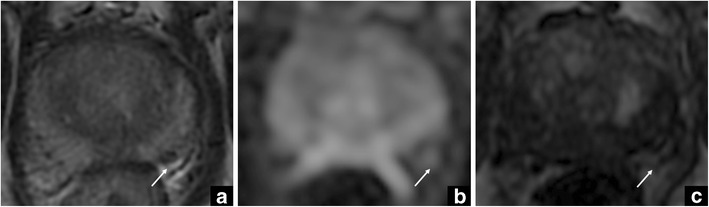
Periprostatic bundle. The arrow in the axial T2-weighted image (a) shows an area of intermediate to low-signal intensity in the right peripheral zone. This corresponds to mild, restricted diffusion on the echo-planar diffusion-weighted sequence (b), due to the slow speed of blood flow and focal enhancement on DCE imaging (c)
